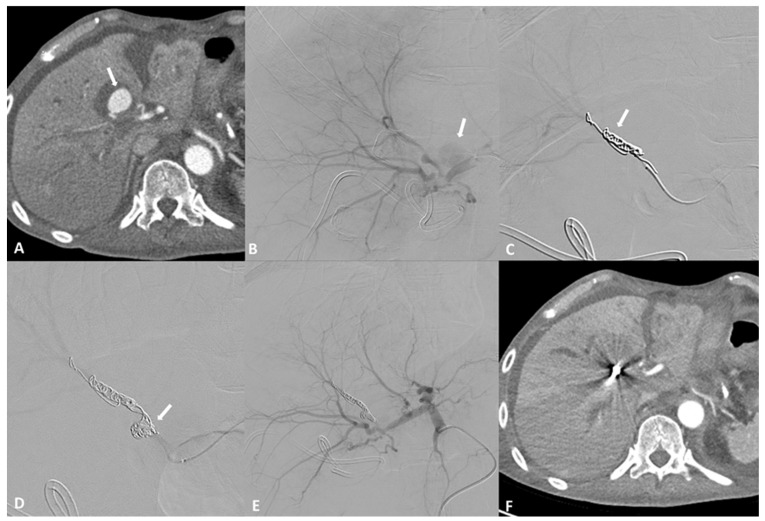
A 50-year-old man with a ruptured hepatic artery pseudoaneurysm (HAP). (A) Contrast-enhanced abdominal CT shows a ruptured hepatic artery pseudoaneurysm (arrow). (B) Selective hepatic angiography demonstrates a pseudoaneurysm arising from the right anterior hepatic artery (arrow). (C) A 5 mm 3D coil is placed in the outflow branch for initial framing (arrow). (D) Additional coils are placed in the focal aneurysmal bulge at the pseudoaneurysm origin (arrow) and the inflow branch. (E) Final angiography demonstrates complete occlusion of the pseudoaneurysm without further contrast extravasation. (F) Follow-up CT at 1 week shows complete embolization of the pseudoaneurysm without evidence of active bleeding.
